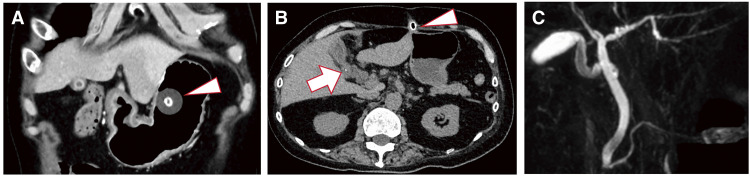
(A and B) Preoperative CT showed a balloon-type gastrostomy in the upper body of the stomach (arrowhead) and a gallbladder stone (arrow). (C) Magnetic resonance cholangiopancreatography showed no morphological abnormalities of the bile duct bifurcation. CT, computed tomography.
